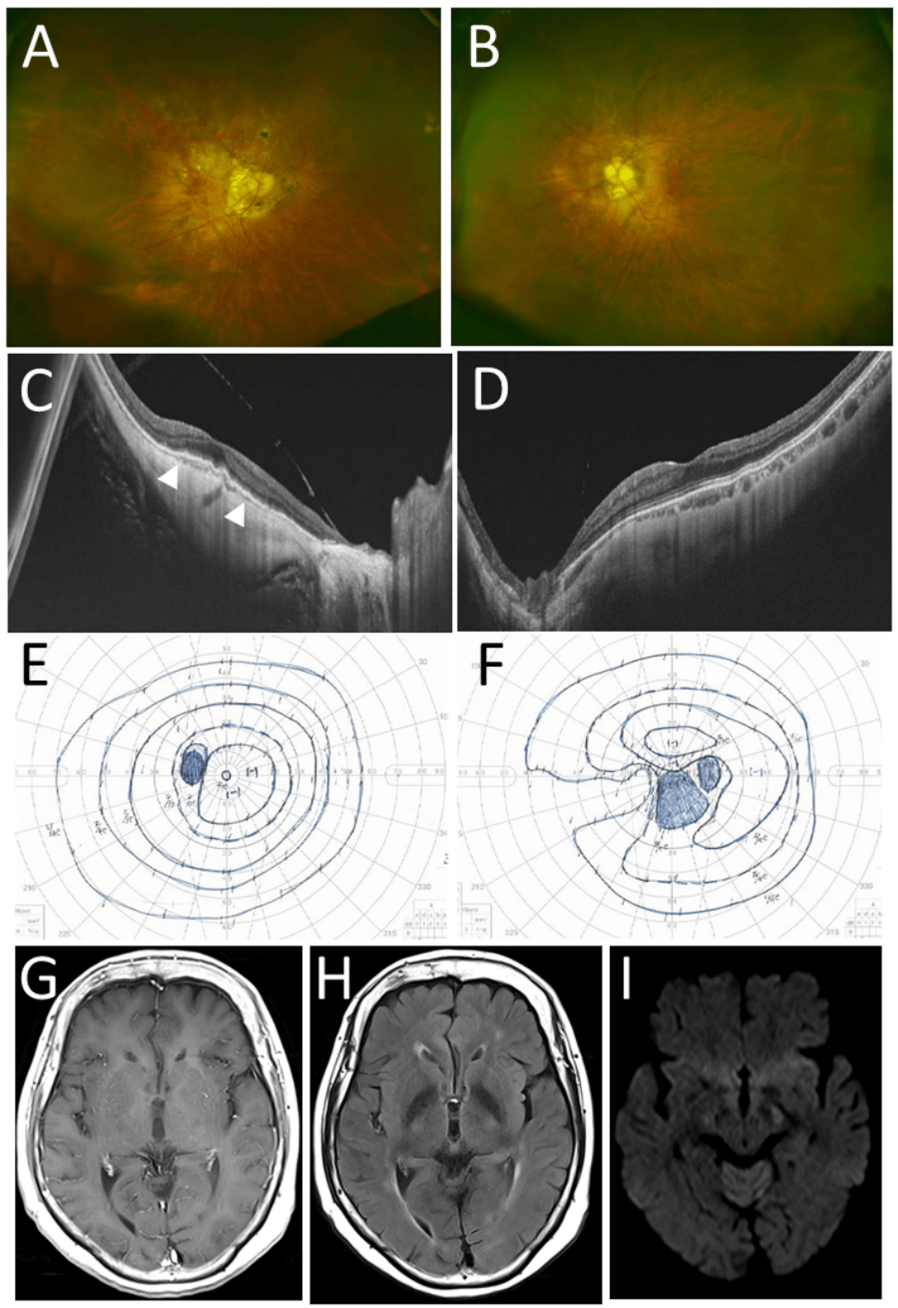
Fundus photographs, optical coherence tomography, Goldmann perimetry, and head magnetic resonance imaging at the age of 75 years Wide-field fundus photographs (A: right eye; B: left eye) and horizontal sections of optical coherence tomography (C: right eye; D: left eye) at the age of 75 years when she noticed a defect in the center of the right vision. Note high-myopic macular degeneration with the loss of the photoreceptor ellipsoid zone (arrowheads) in the right eye, the same as Figure 1C. Goldmann perimetry (E: left eye; F: right eye) showing central scotoma which deviates inferiorly in association with a glaucomatous nasal rupture-like defect (F) in the right eye. Magnetic resonance imaging (G: T1-weighted image with contrast enhancement; H: T2-weighted FLAIR image; I: diffusion-weighted image) showing no lesion, compared with the left occipital lesion a year later (Figure 3A, 3B). FLAIR: fluid-attenuated inversion recovery
